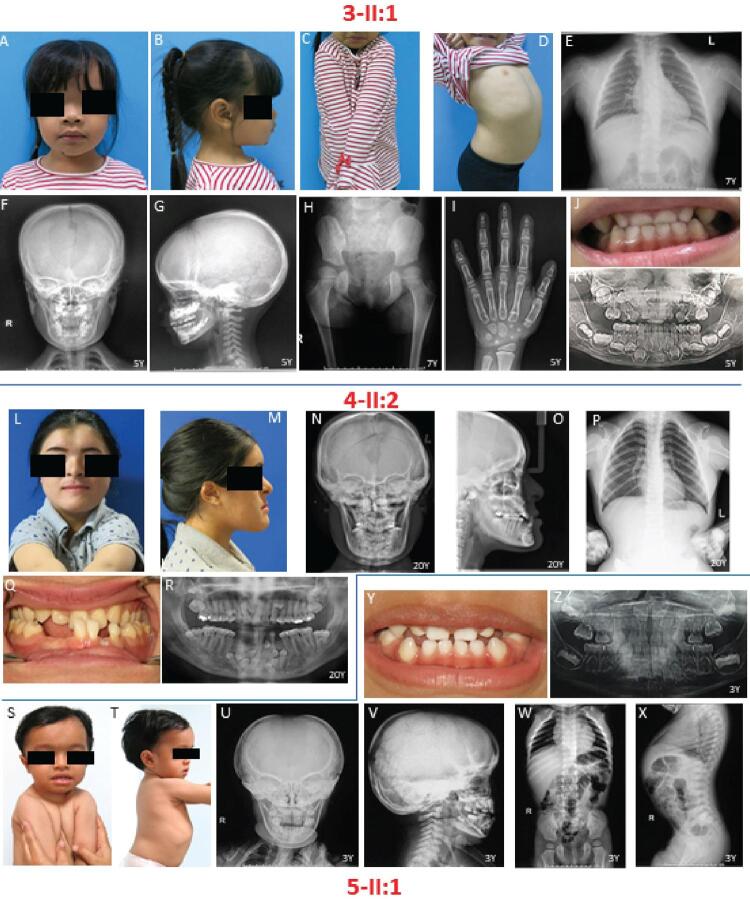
Clinical and radiographic features of 3-II:1, 4-II:2, and 5-II:1. (A-K) 3-II:1 shows macrocephaly and lordosis. Radiographs shows aplastic clavicles, a “chef’s hat” appearance of the femoral head, partial pseudoepiphysis of 2
